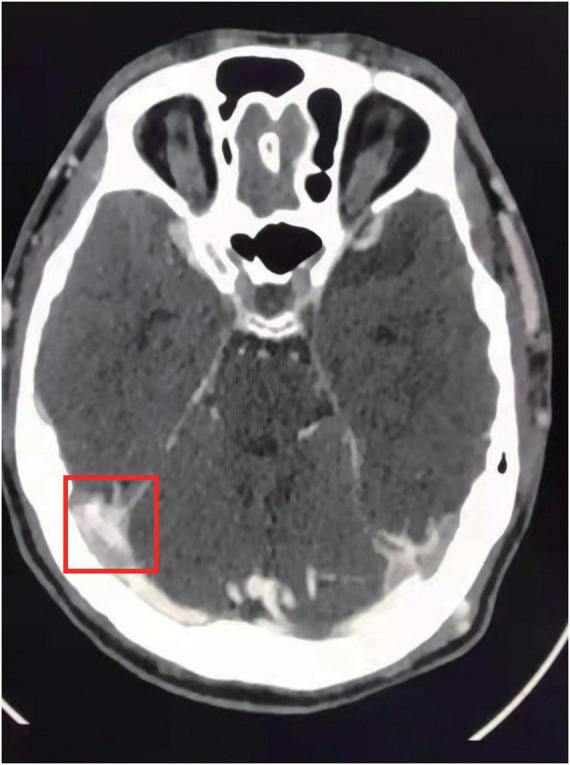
MRI scan indicated dural sinus thrombosis.
